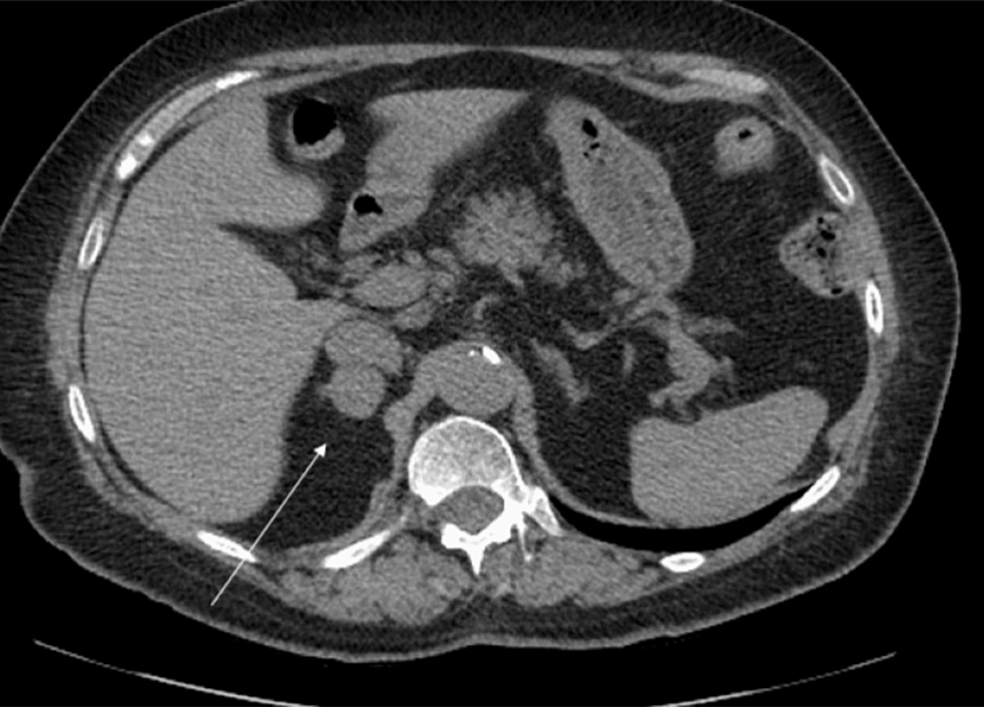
CT abdomen with a hypodense lesion in the right adrenal gland CT: computed tomography
